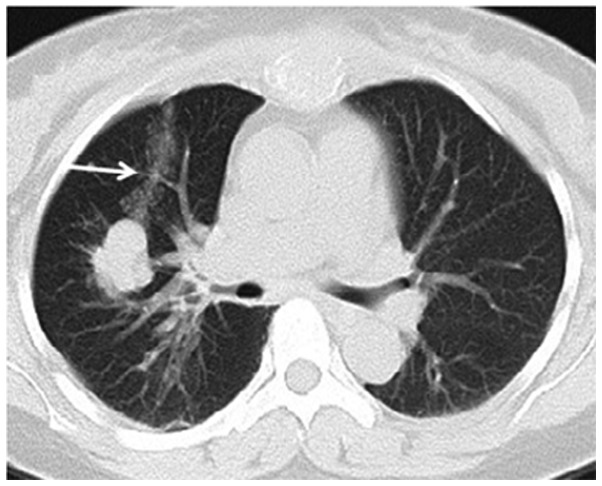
CT scan image. Mild hemorrhage with haziness along the needle tract.
